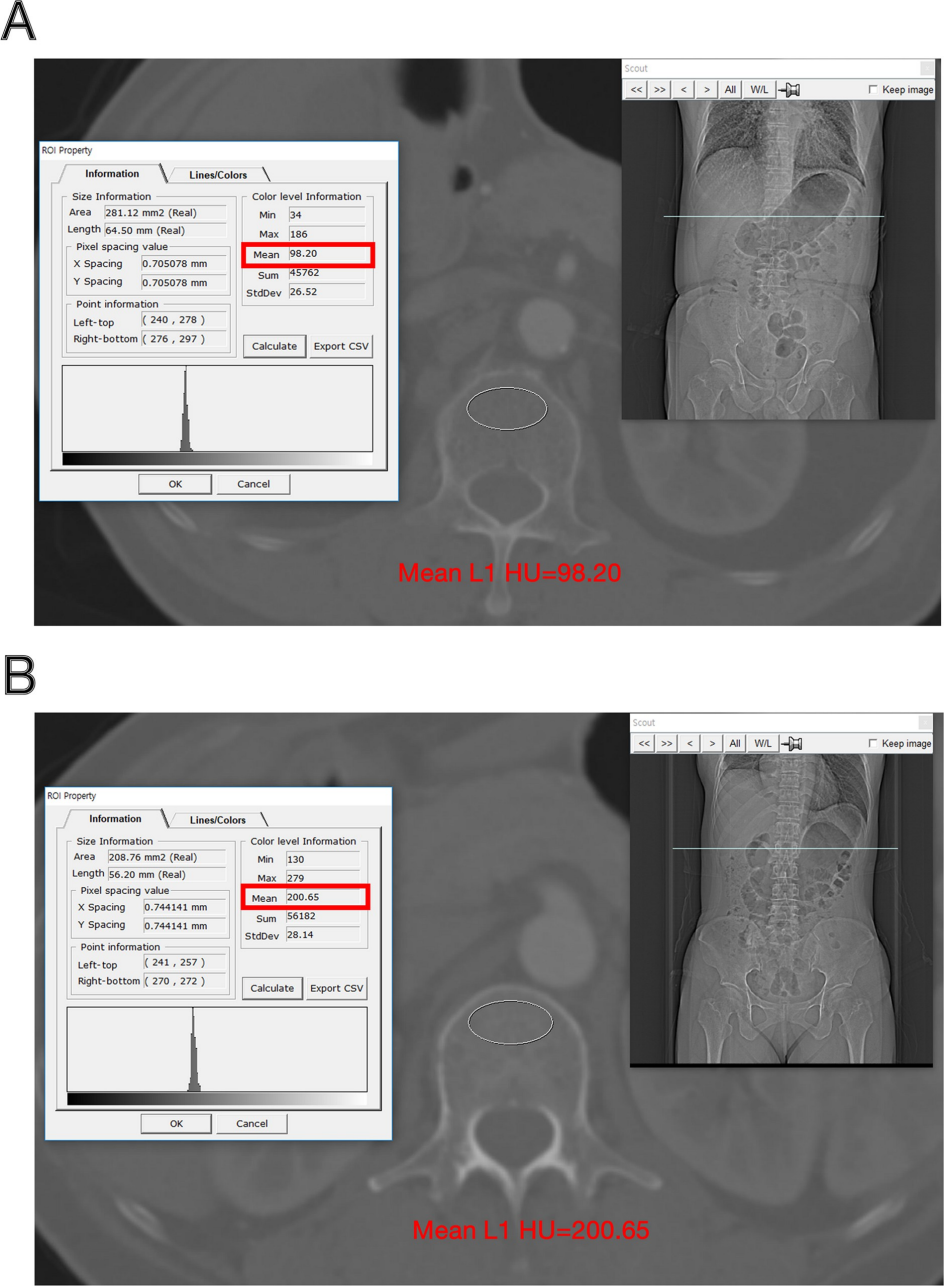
Examples of measurement of HU values in the trabecular bone of the middle of the L1 vertebral body. The PACS automatically calculates the mean HU value according to ROI line, and the mean HU value is recorded. (A) CT showing relatively low mean L1 HU value; (B) CT showing relatively high mean L1 HU value. HU = Hounsfield unit; PACS = picture archiving and communication system; ROI = region of interest.
